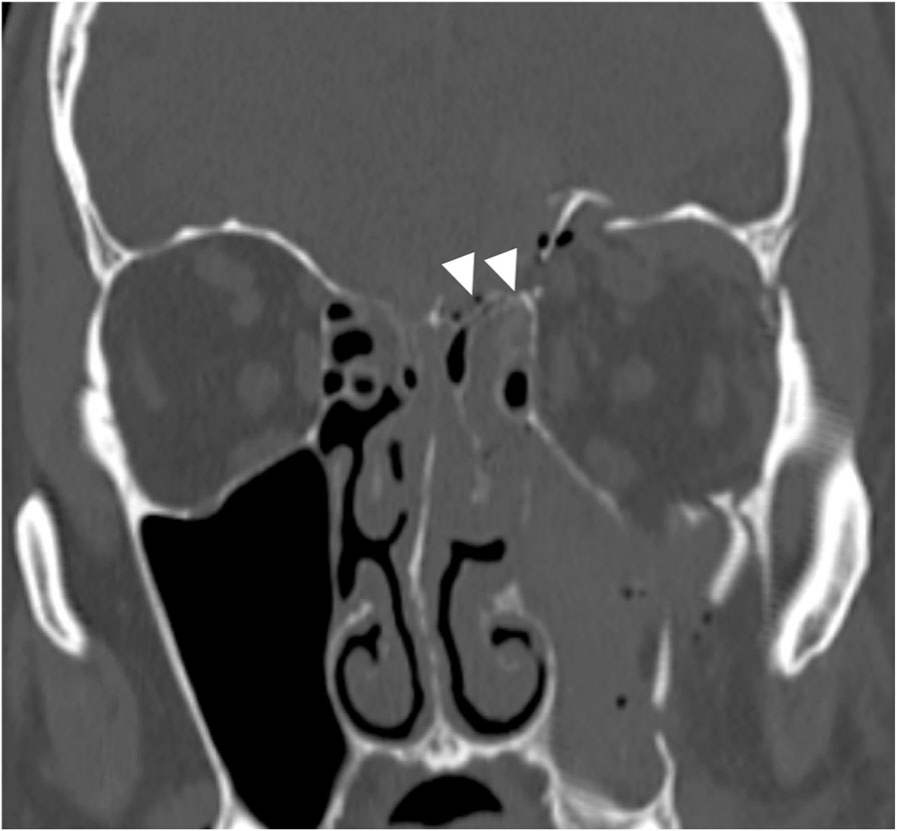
Fracture of cribriform plate. CT coronal image shows multiple fractures of the skull base. Note in particular the fracture of the cribriform plate (arrowheads)
